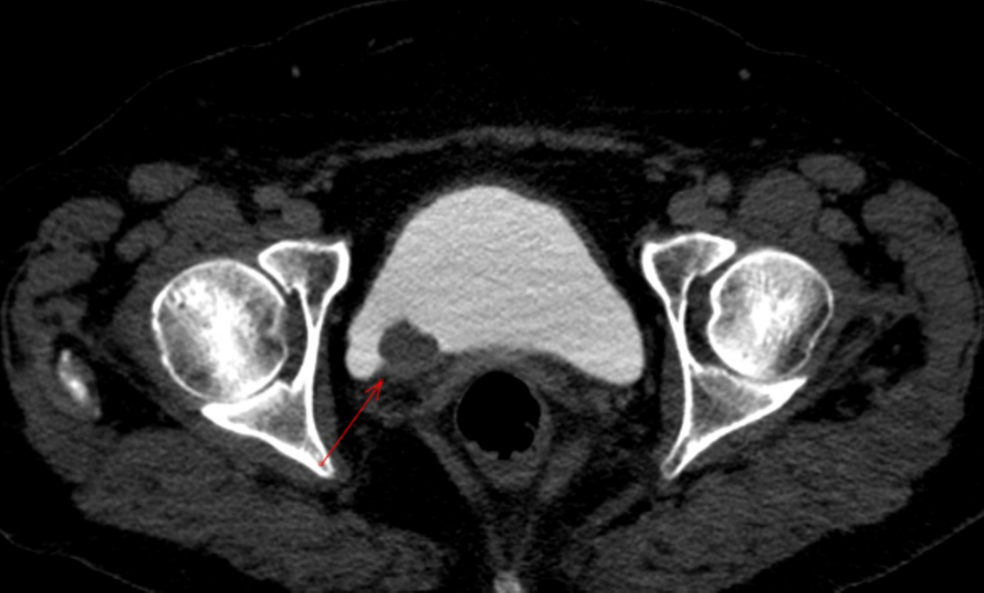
Axial computed tomography view of the tumor in the bladder (red arrow pointing to the tumor)
